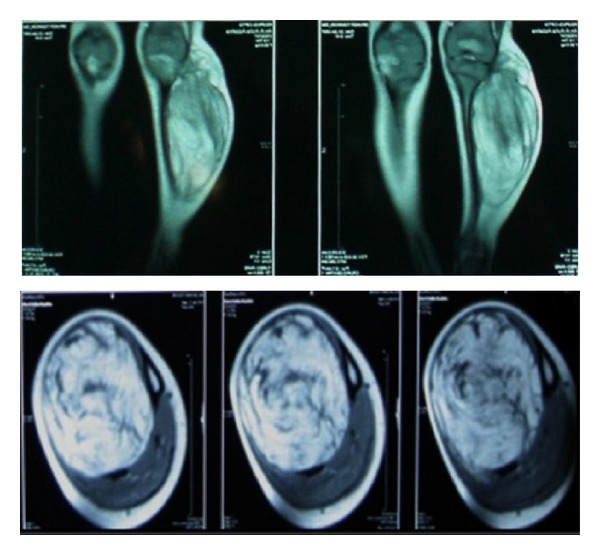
MRI of Case  1 in coronal and axial view of T1-weighted images. The soft tissue mass showed predominantly lipomatous tissue with some part of non-fatty component. The lession was well-circumscribed, with compression of adjacent bones and muscles.
